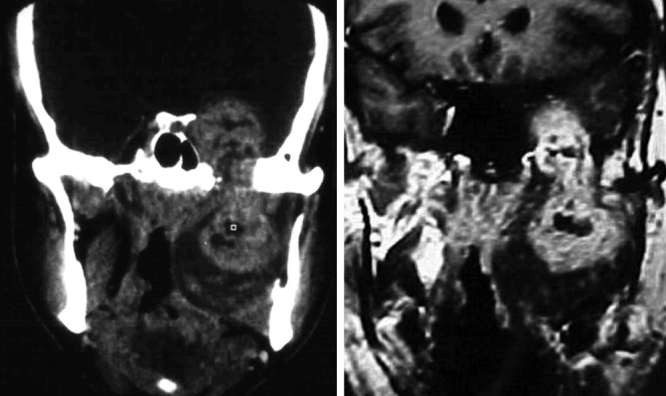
Mandibular nerve schwannoma: coronal computed tomography and magnetic resonance imaging show a hourglass-like mass with a localized shaft and widening of the oval foramen, with intracranial components and in the masticator space.
